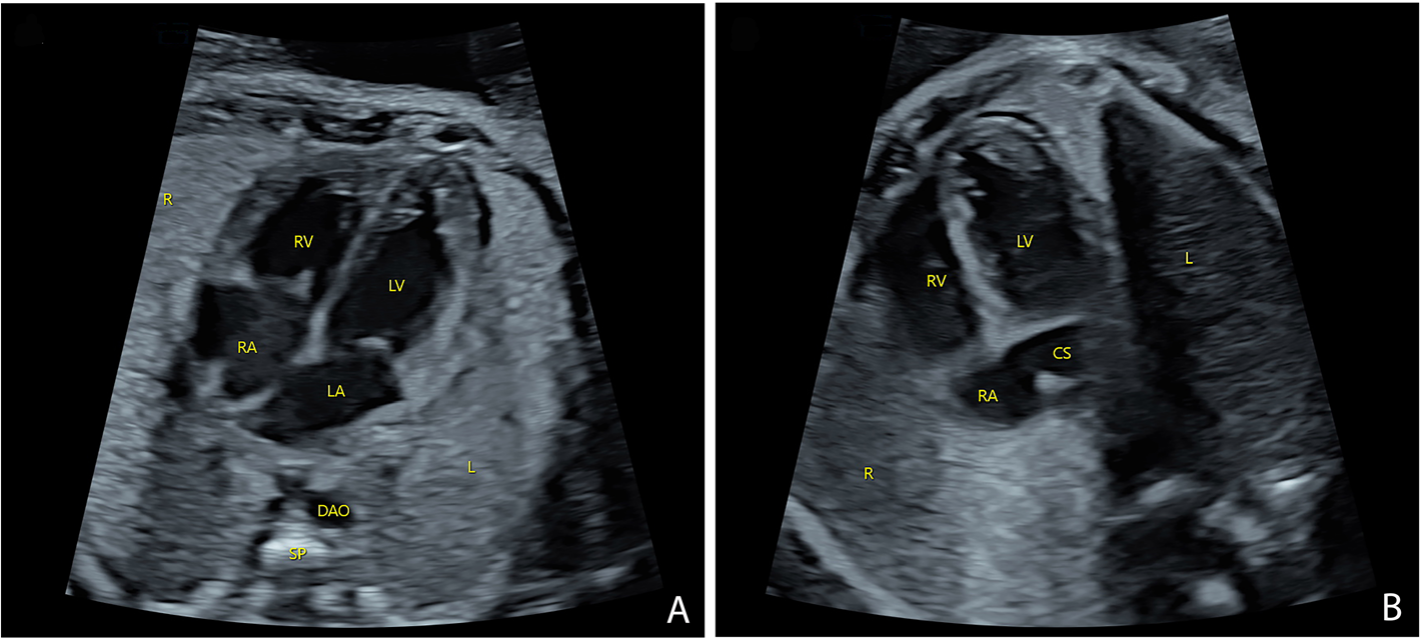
Sonograms showing the symmetrical four chambers (a) and the dilated coronary sinus (b). CS: coronary sinus; DAO: descending aorta; L: left; LA: left atrium; LV: left ventricle; R: right; RA: right atrium; RV: right ventricle; SP: spine
